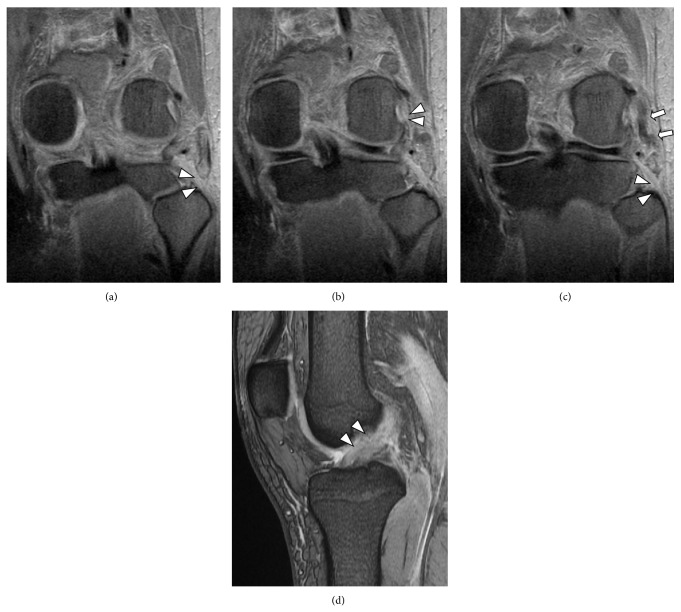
(a) Magnetic resonance imaging (MRI; T2 weighted coronal image) showing the absence of the biceps femoris tendon (arrow head). (b) MRI (T2 weighted coronal image) showing the popliteus muscle tendon rupture at the femoral attachment (arrow head). (c) MRI (T2 weighted coronal image) showing the lateral collateral ligament (LCL) rupture at the fibula head (arrow head) and substance of the LCL (arrow). (d) MRI (T2 weighted sagittal image) showing the anterior cruciate ligament rupture (arrow head).
